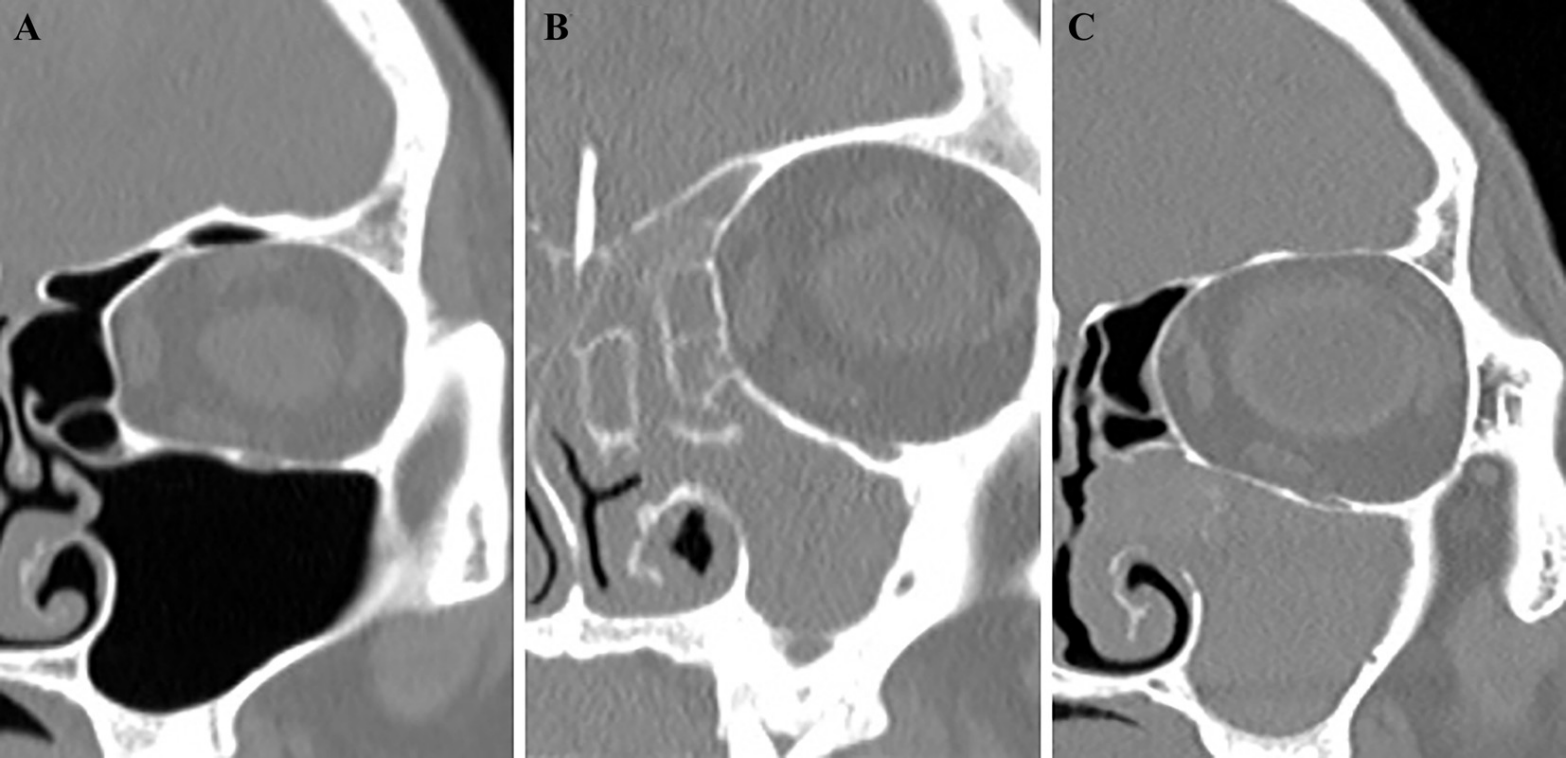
Coronal computed tomography images of representative cases with (A) healthy control, (B) chronic rhinosinusitis, and (C) maxillary sinus fungal ball on half coronal CT slices.
